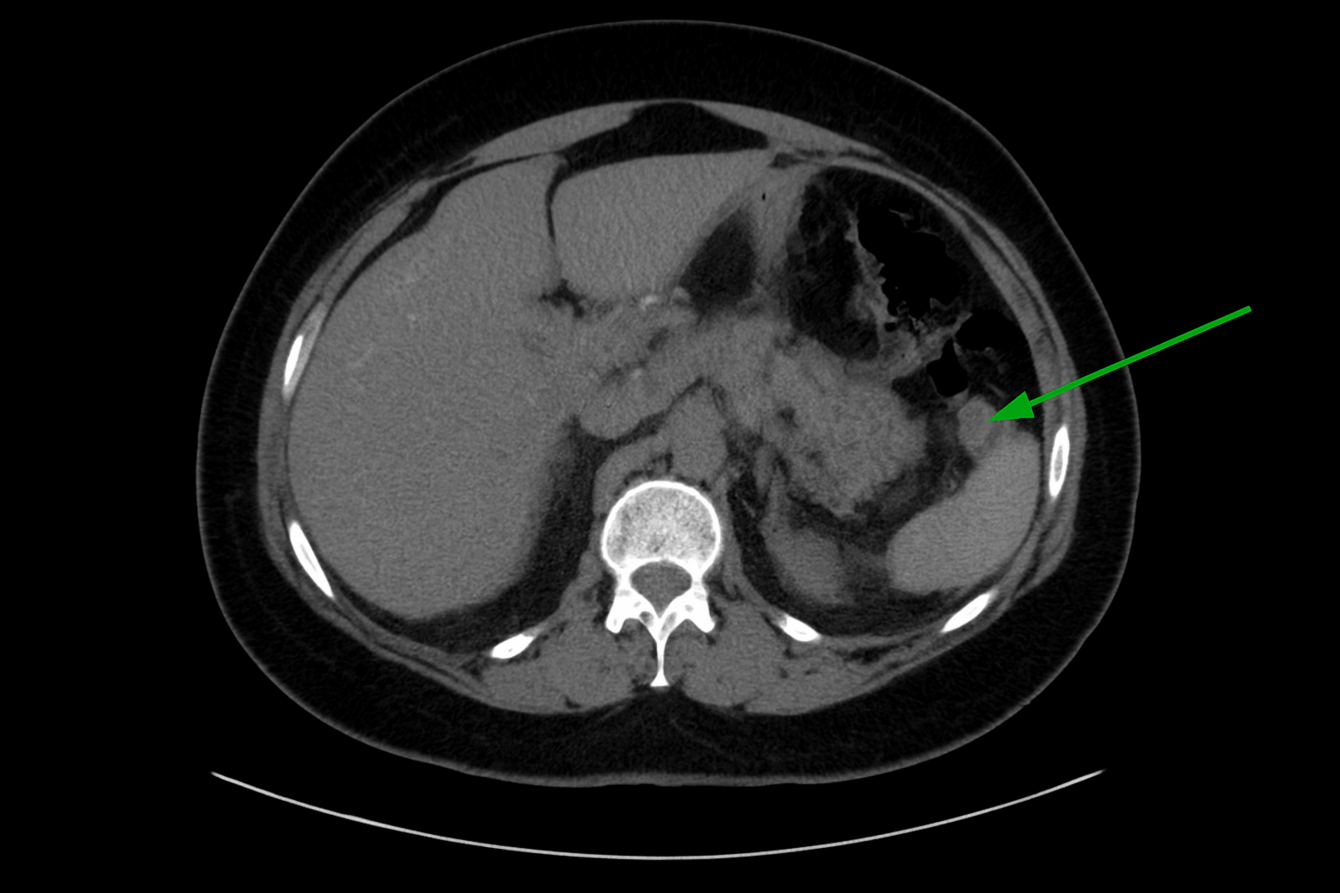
Non-contrast-enhanced computed tomography scan of the abdomen The green arrow indicates the site of fat stranding. Source: Radiology department, Port of Spain General Hospital
